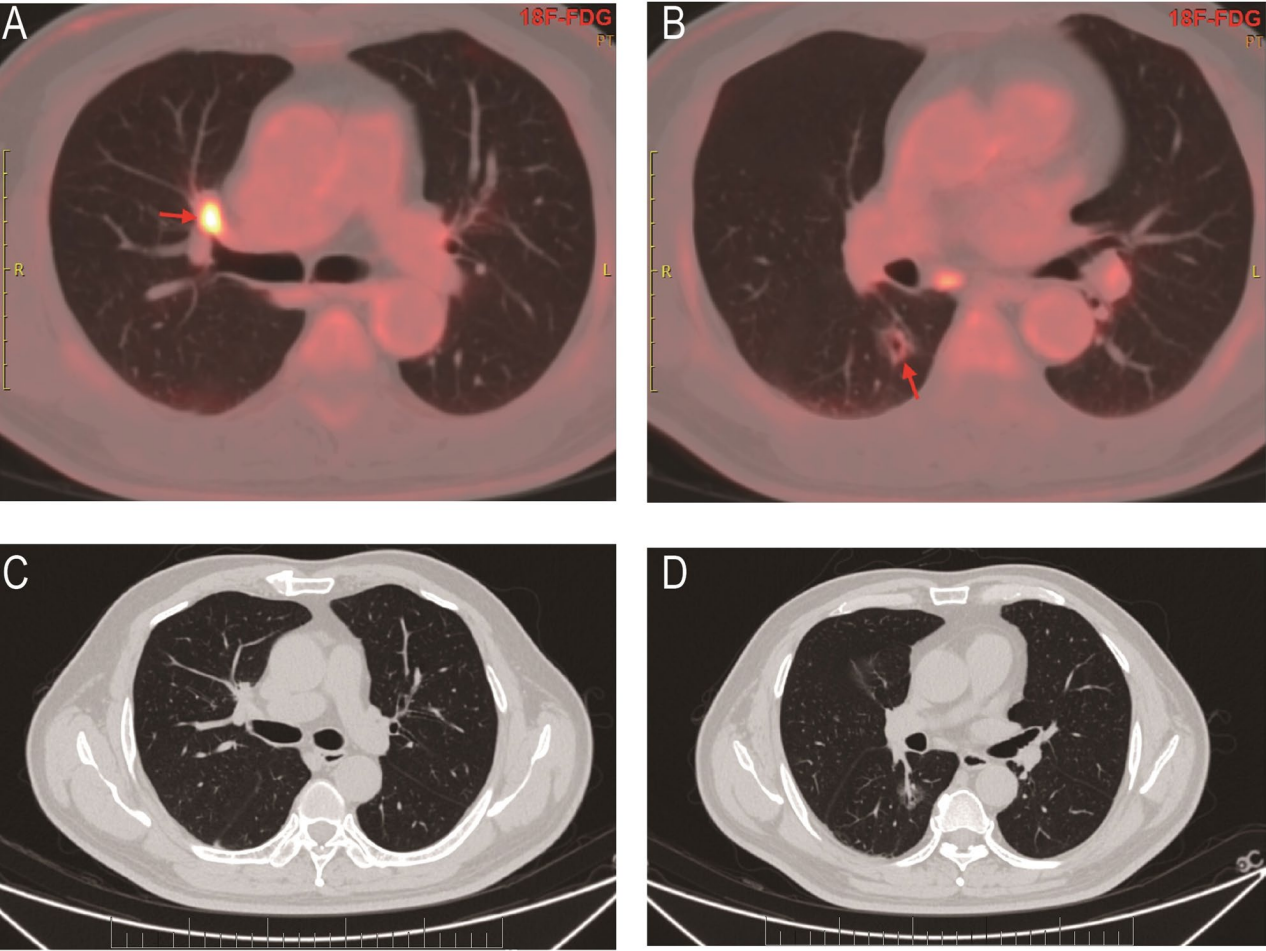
Preoperative PET-CT shows synchronous primary lung cancers. (A, C) A hypermetabolic tumor in the RS3 segment of the right upper lobe. (B, D) A separate primary tumor presenting as mixed-density nodules with mild hypermetabolism in the RS6 segment of the right lower lobe
